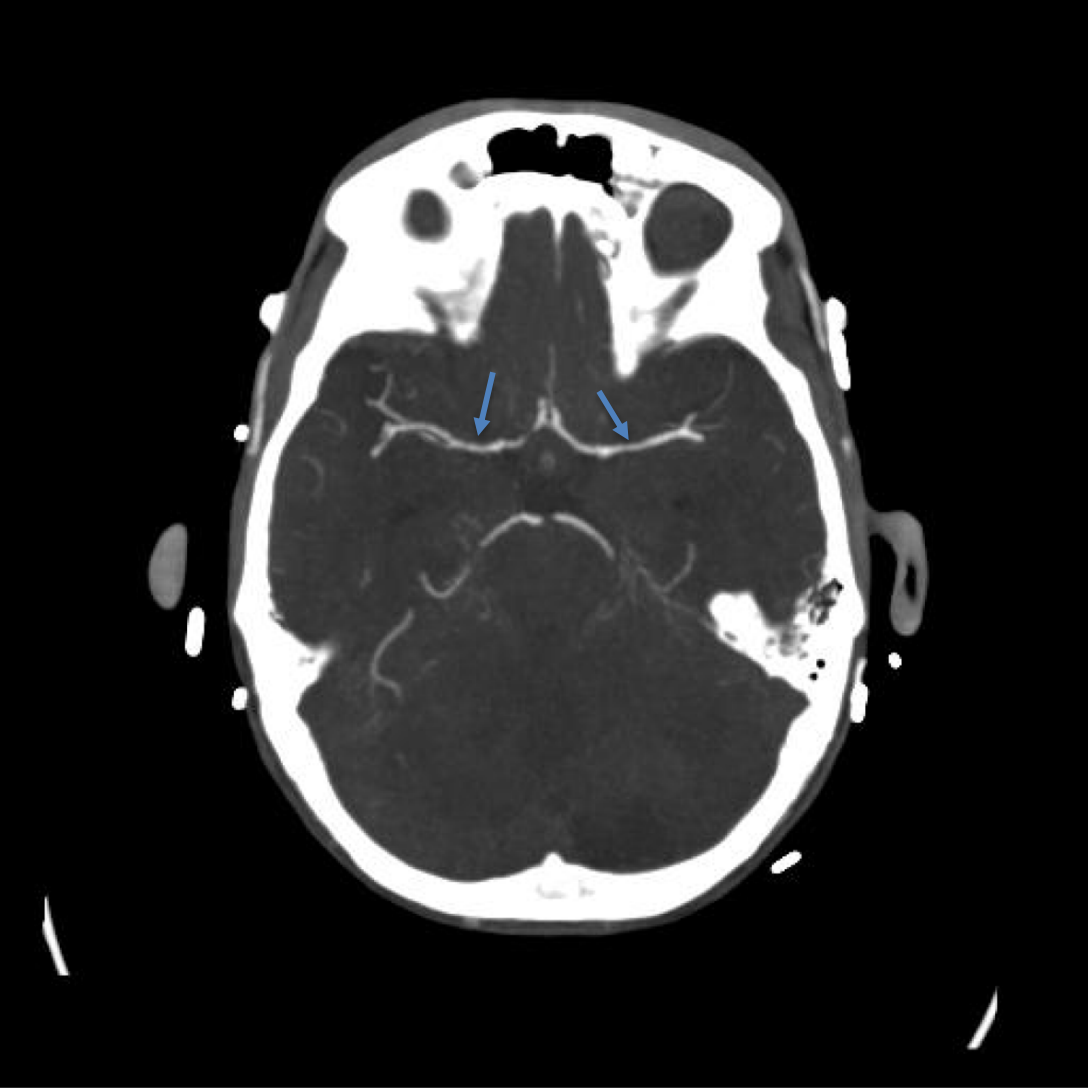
CT Angiogram of brain case 2 revealing irregular narrowing of the M1 MCA consistent with a vasculitis
